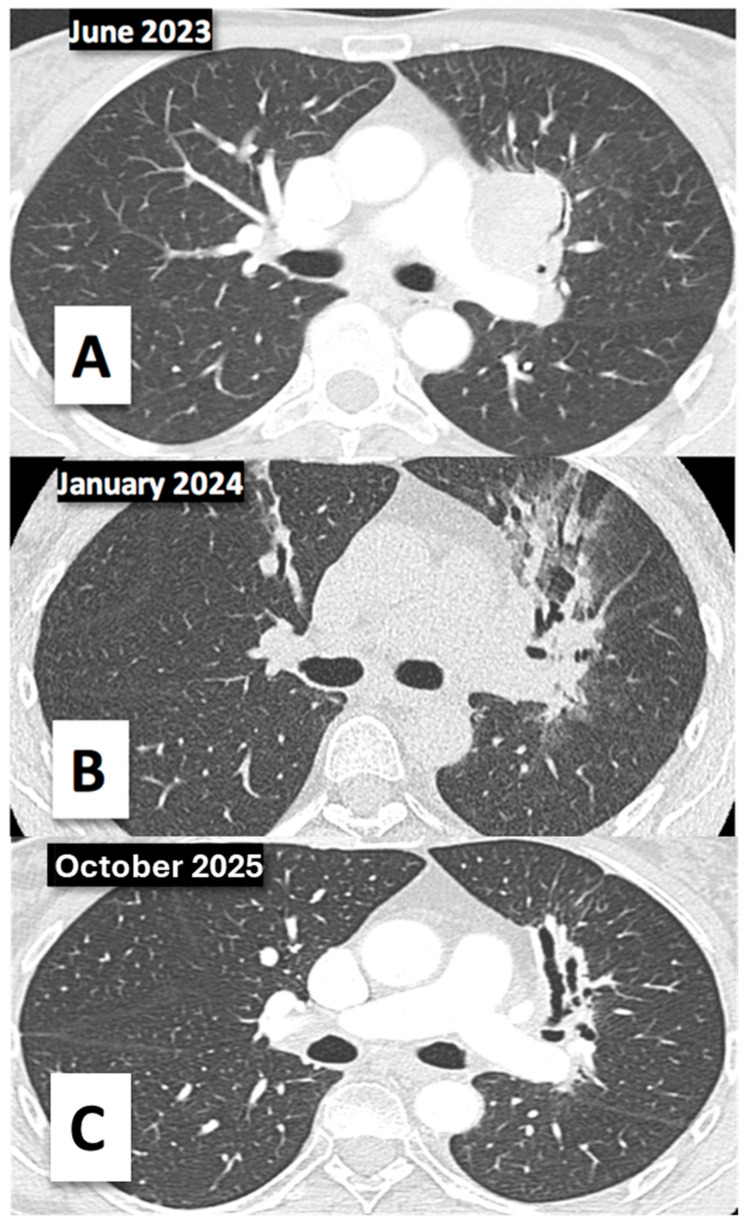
(A) Squamous cell lung carcinoma T4N3M0. Stage IIIC. Diagnosis in June 2023. (B) High resolution CT scan in January 2024. Peribronchial consolidation and post-radiotherapy pneumonitis and (C) complete response maintained in October 2025, with residual signs of post-radiotherapy pneumonitis.
